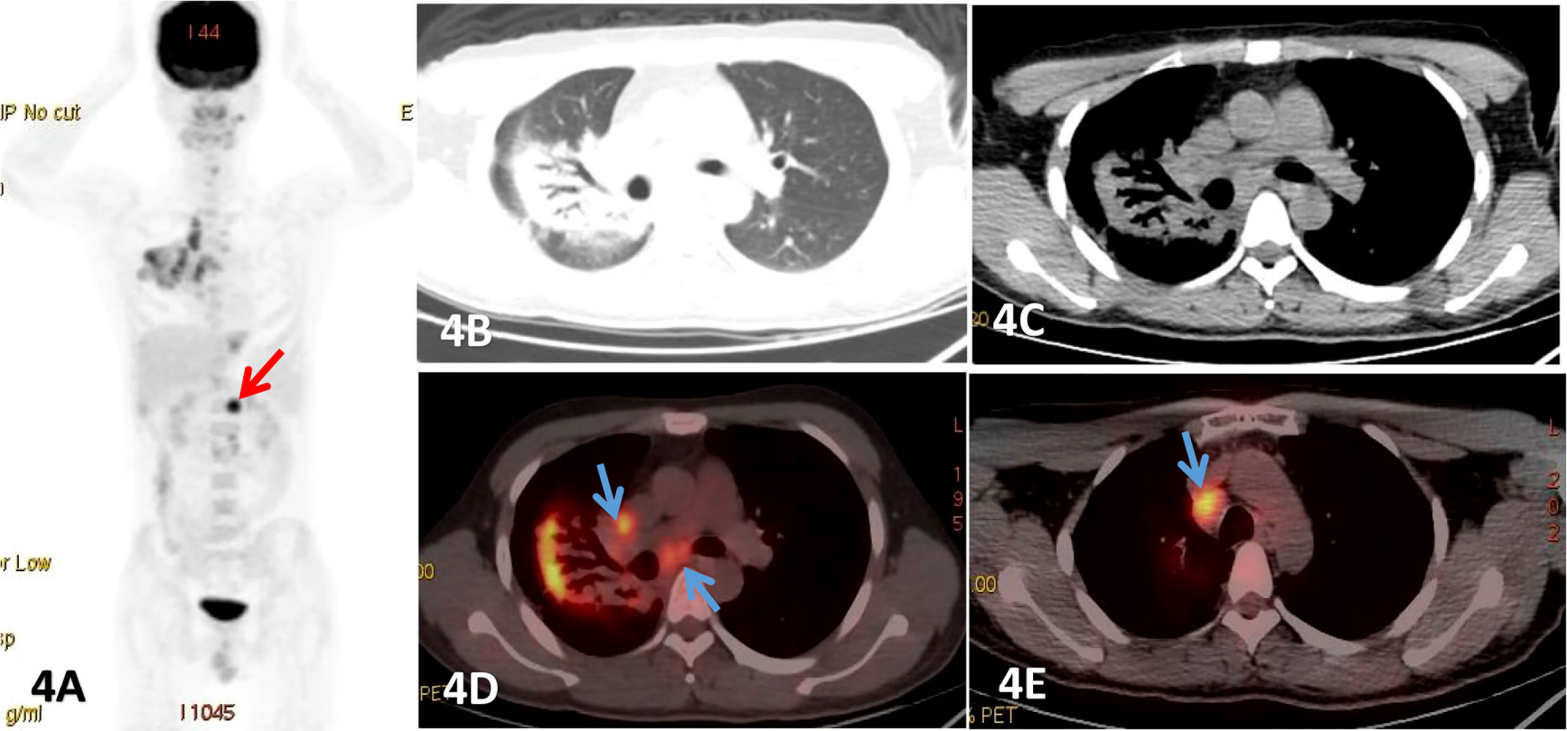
Male, 25 years old, fever, cough, dyspnea for more than 2 months, (A) was PET MIP image, (B, C) were lung and mediastinum CT transection scan, (D, E) were PET/CT fusion image of mediastinum.Patchy consolidation shadows were observed beside the right hilar, and obvious dilated bronchial shadows were observed in the lesions, presenting a translobular distribution with clear margins, which FDG uptake increased with a SUVmax of 8.52, and lung MALT lymphoma was detected by fiberbronchoscopy biopsy. And an enlarged lymph node with significant uptake of FDG was also seen below the diaphragm (red arrow). Endoscopic ultrasonic-guided transbronchial needle aspiration.
